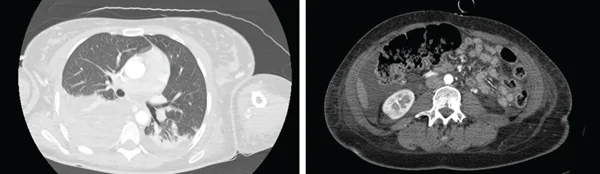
Thoracic and abdominal CT scan showing right pleural and peritoneal effusion.
